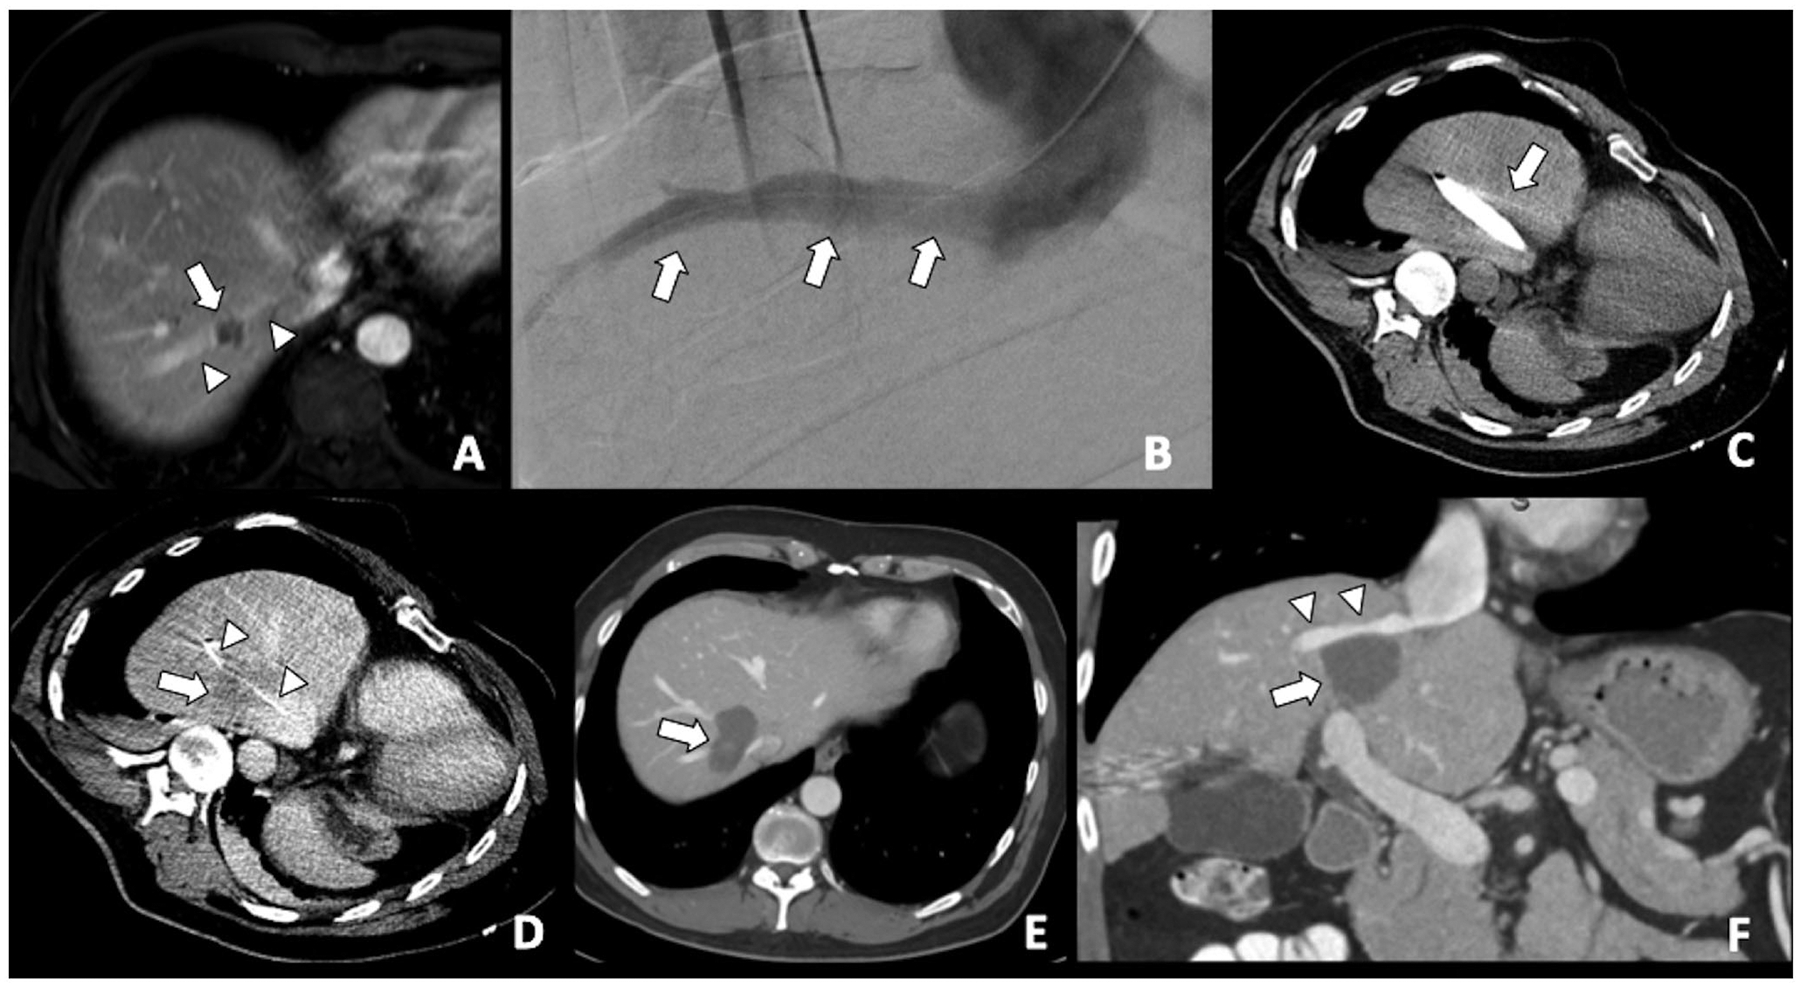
A colorectal liver metastasis abutting right hepatic vein treated by combination of percutaneous transhepatic venous temporary balloon occlusion of the right hepatic vein and microwave ablation to compensate the heat-sink phenomenon. (A) Magnetic resonance imaging demonstrating a tumor (arrow) abutting right hepatic vein (arrowheads); (B) Percutaneous transhepatic hepatic venography demonstrating the right hepatic vein (arrows); (C) Intra-procedural CT depicting inflated balloon catheter in the right hepatic vein during ablation; (D) A Clear ablation zone (arrow) covering the right hepatic vein, which is indicated by the catheter (arrowheads); (E) One month follow-up CT revealing satisfactory ablation zone (arrow) without residual tumor; (F) CT coronal multiplanar reconstruction demonstrating the ablation zone (arrow) and patent right hepatic vein (arrowheads).
